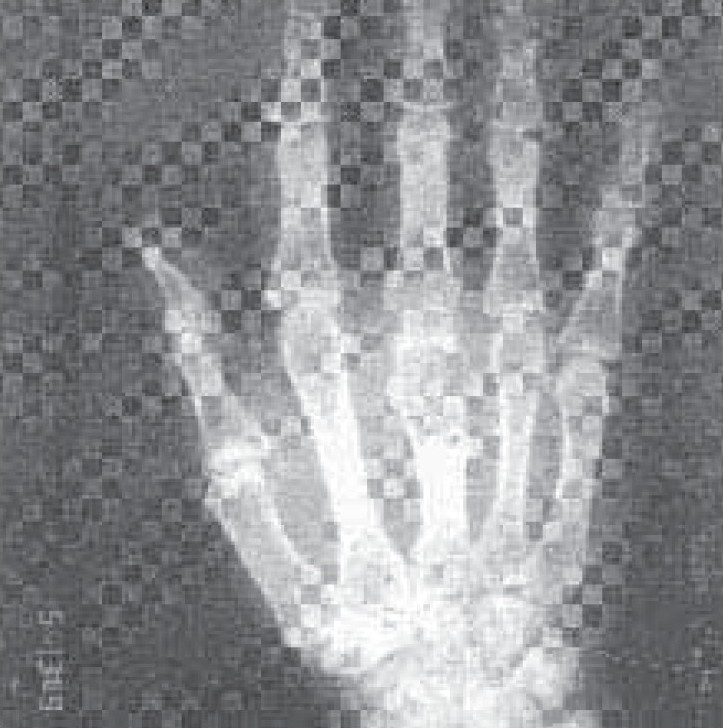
X-ray Left wrist and hand showing lytic lesions over head of third metacarpal bone.
